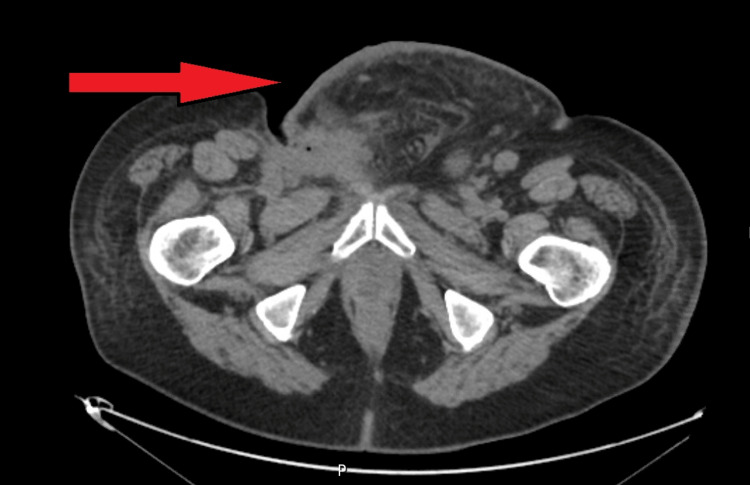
Abdominal CT image showing the tumoral pubic mass
